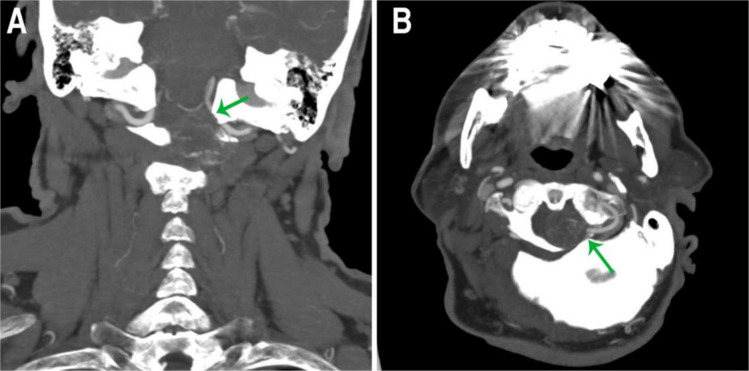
CTA views of the lesion demonstrating its close relationship with the V3 segment of the vertebral artery (green arrows). A Coronal view. B Axial view
